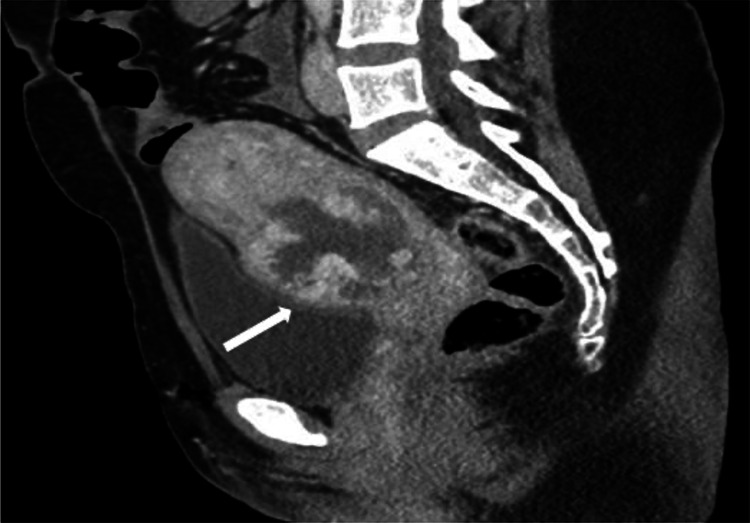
CT of sagittal section of the pelvis, highlighting the abnormal location and heterogeneity of the pregnancy. The arrow highlights the distinct uterine outpouching toward the bladder, which is common in cesarean scar ectopic pregnancies, and may indicate invasion into nearby structures.
